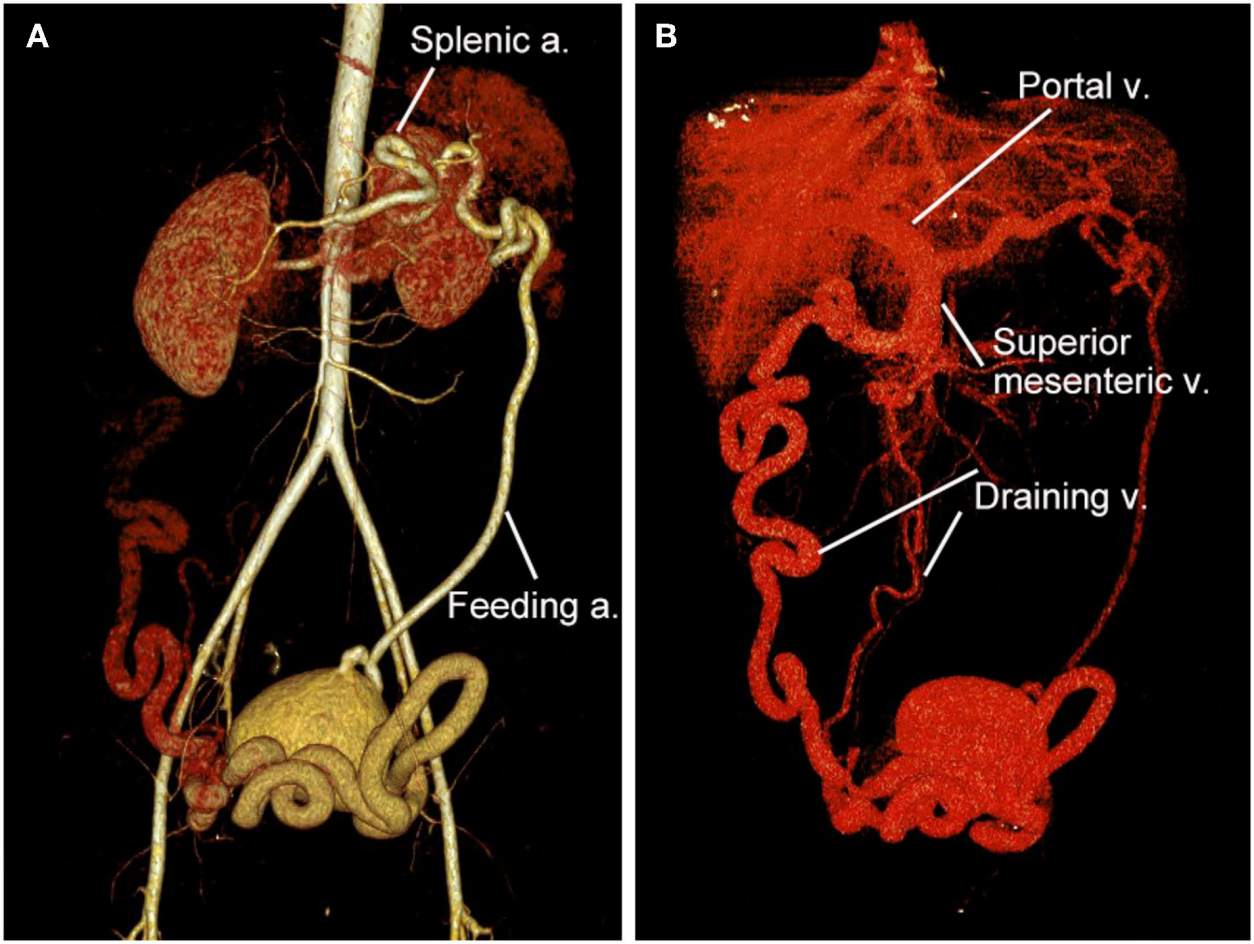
3D reconstruction of CT angiography of the abdominopelvic arteriovenous malformation (AVM). (A) Arterial phase of CT angiography showed the AVM had a single feeding artery originating from the splenic artery. (B) Venous phase of CT angiography showed the AVM had two draining veins into the superior mesenteric vein and then the portal vein.
